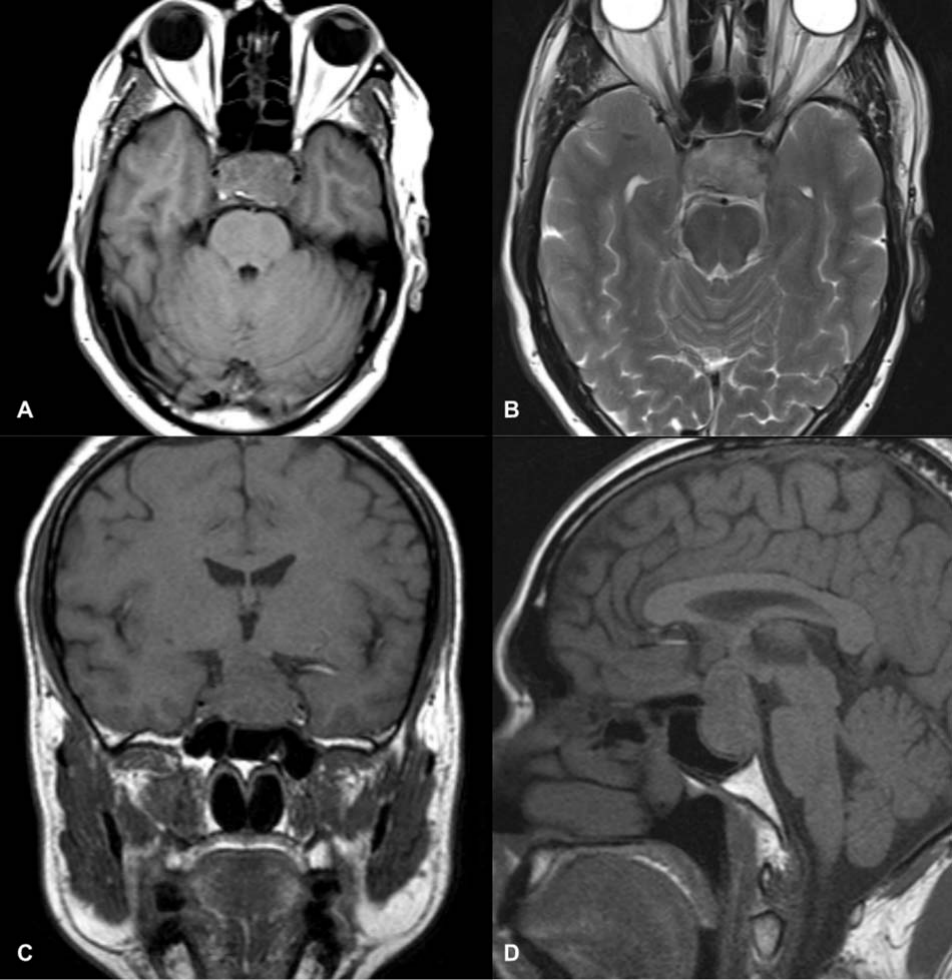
MRI images of axial T1 (A), axial T2 (B), coronal T1 (C), and sagittal T1-weighted images (D) showing a 3 cm × 2.9 cm × 1.8 cm sized suprasellar lesion displacing optic chiasma superiorly and laterally causing a mass effect on bilateral cavernous sinuses (Left>Right) with patchy areas of high signal on T1-weighted images and signal void on T2-weighted images suggestive of hemorrhage.
